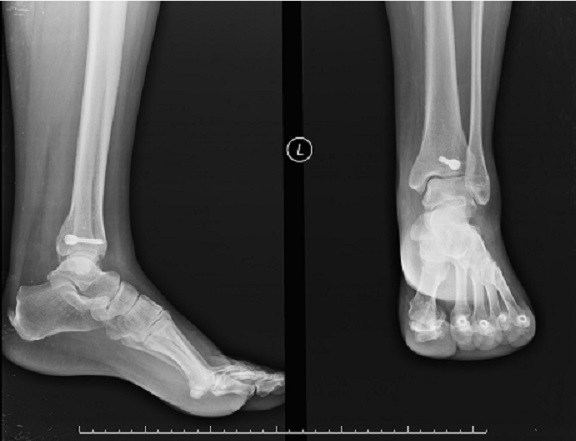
On final radiographies, union was verified
